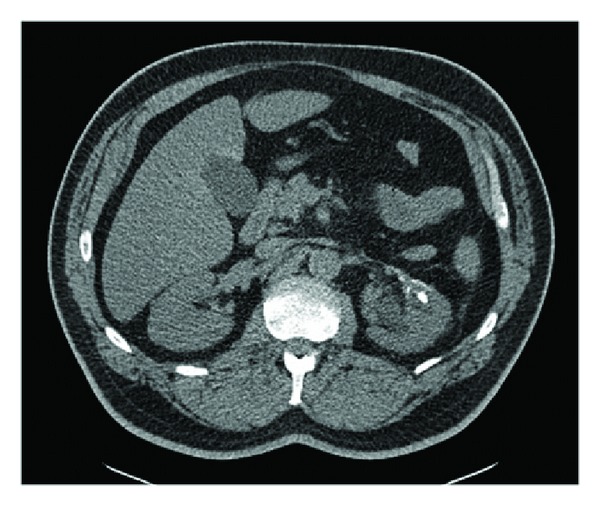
Noncontrast helical abdominal CT scan showing persistent 10 mm left renal calculus after extracorporeal shock wave lithotripsy.
